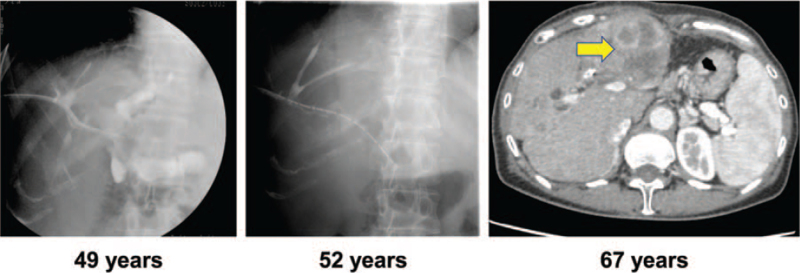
Few bile ducts were visible on cholangiography. In a contrast-enhanced CT scan, an intrahepatic cholangiocarcinoma was visible in the residual left lobe of the liver.
